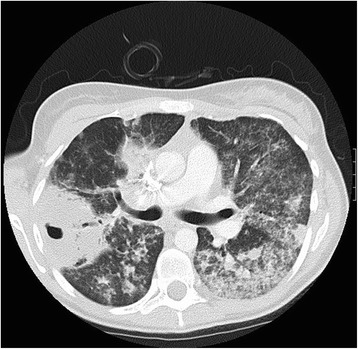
High-resolution chest computed tomography in a 16-year old girl affected by granulomatosis with polyangiitis (GPA). The image shows multiple nodules and regions of consolidation of variable size, irregularly marginated with peribronchovascular distribution. Cavitations are demonstrated in several nodules, the largest in the right lung (6.5×4.5 cm). Diffuse alveolar opacities are consequence of haemorrhages
